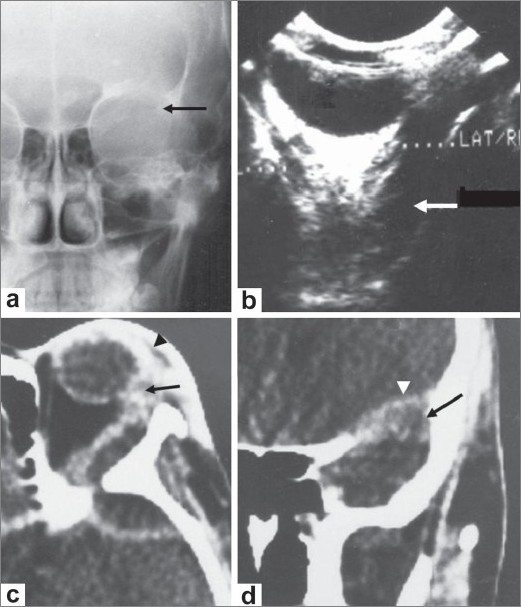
A 11-year-old girl presented with painless, nonpulsatile proptosis of the left eye of 3 months' duration. Caldwell view radiograph (a) shows destruction of the greater wing of the sphenoid on the left side
